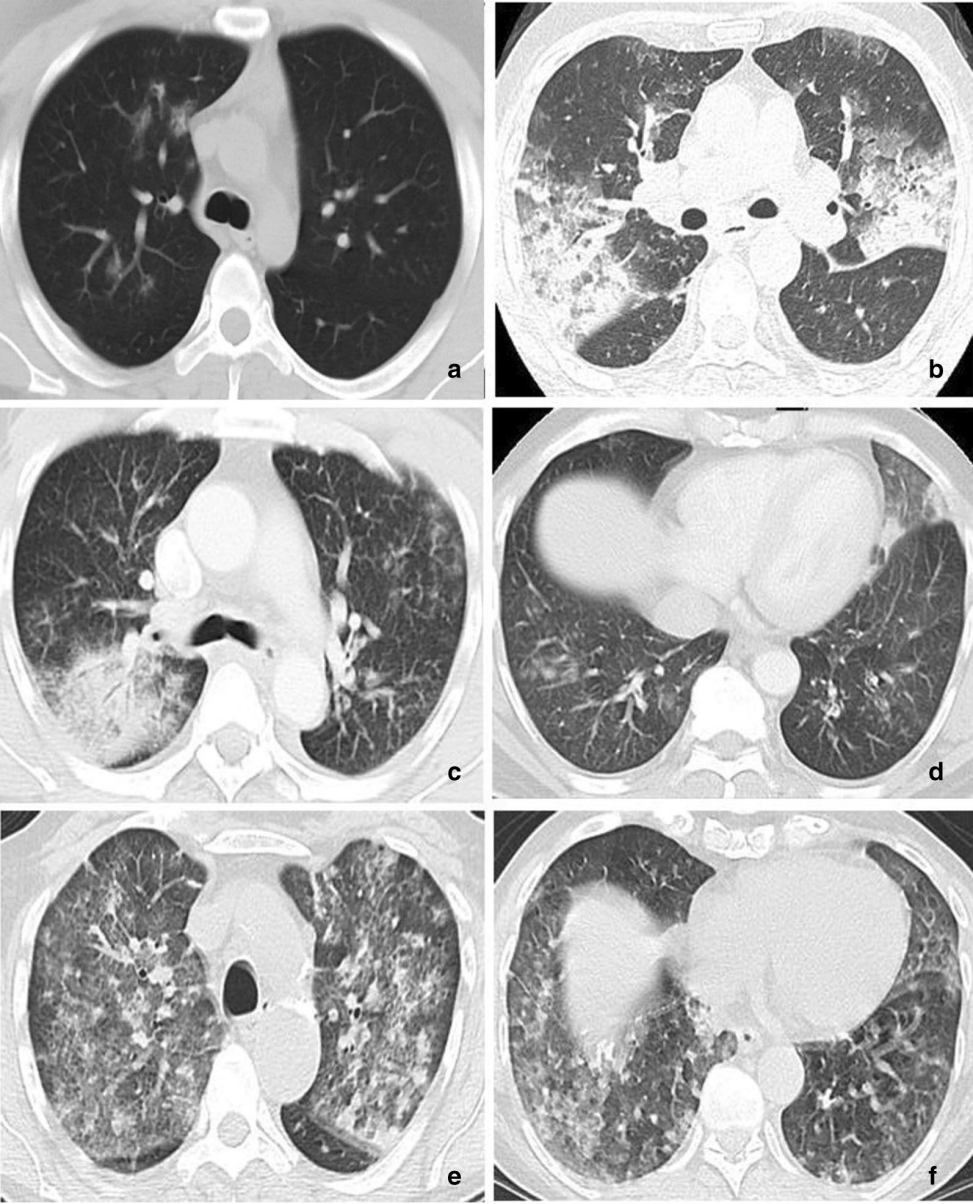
Indeterminate COVID-19 and viral pneumonia mimickers. a, b Indeterminate CT features for COVID-19 in two different patients with positive RT-PCR test, axial CT images show unilateral small areas of non-peripheral GGO (a) and bilateral multifocal non-rounded GGO of non-specific distribution (b). c, d Adenovirus pneumonia in a 48-year-old man, axial CT images show bilateral area of consolidation with nearby GGO and bilateral centrilobular nodules. e, f HSV pneumonia in a 75-year-old woman, axial CT images show widespread bilateral GGO of non-rounded morphology, small areas of consolidation and diffuse interlobular septal thickening
